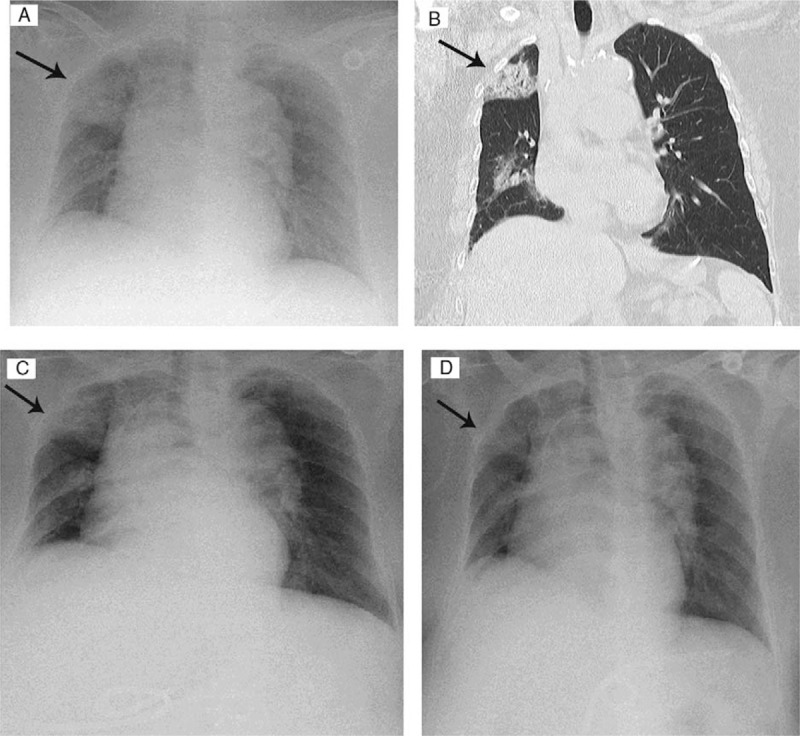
Patient No. 14 had incidentally discovered patchy pneumonia (black arrows) over the right upper lobe (A, chest X-ray; B, chest computed tomography), which had poor response to 5 days of antibiotic treatment (C). Serial images indicate disease resolution following 8 days of methylprednisolone treatment (D).
